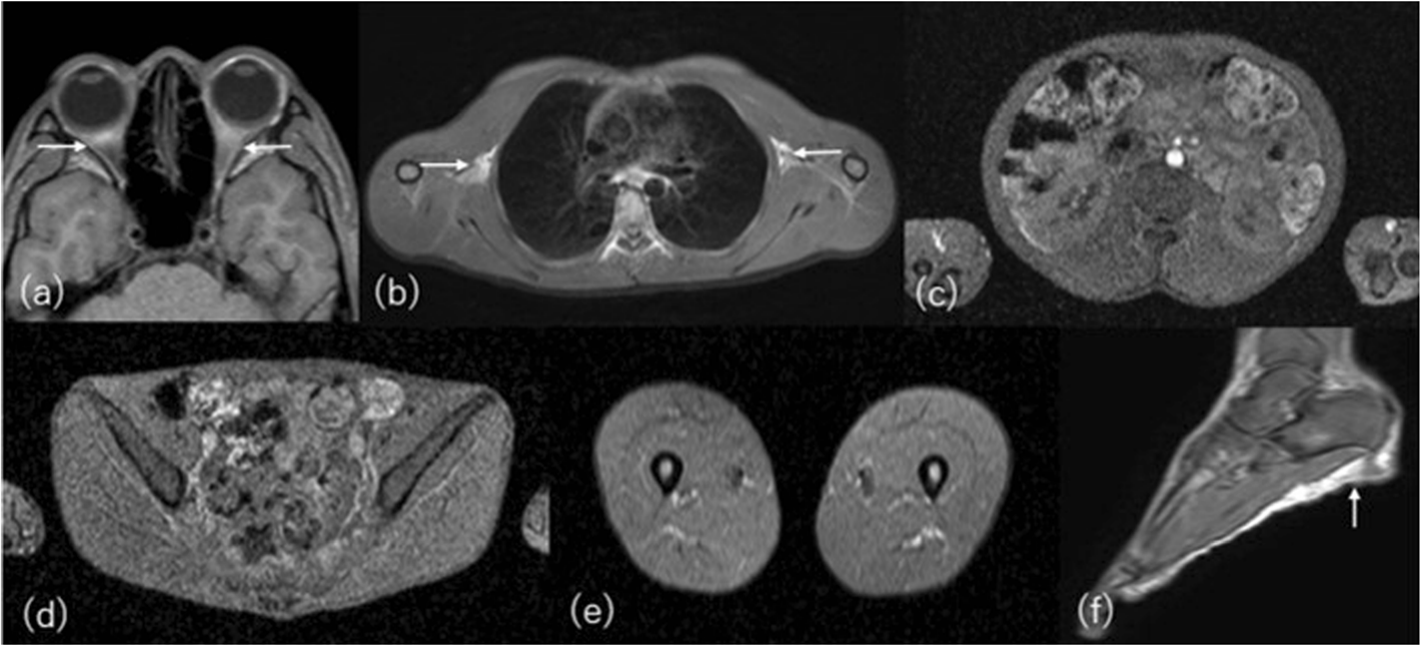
Whole body MRI taken from the same CGL patient 10 years earlier. T1-weighted axial images at the level of (a) orbita, (b) axilla, (c) renal sinus, (d) pelvis, (e) thigh, and (f) sagittal image of foot show nearly complete absence of adipose tissue, which is however slightly preserved in the orbits, axillae, and soles (arrows)
